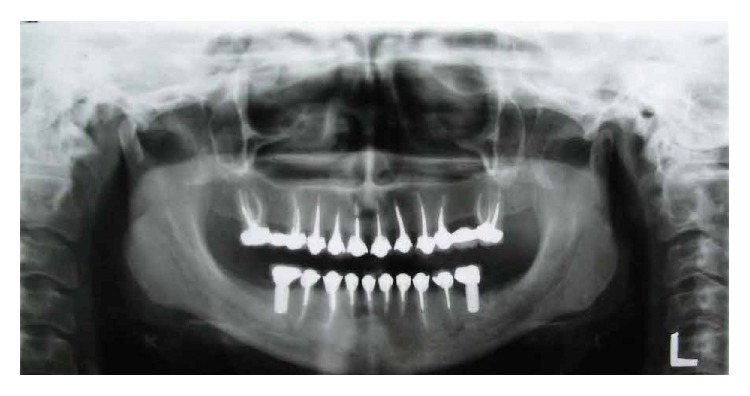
Posttreatment panoramic radiography.
